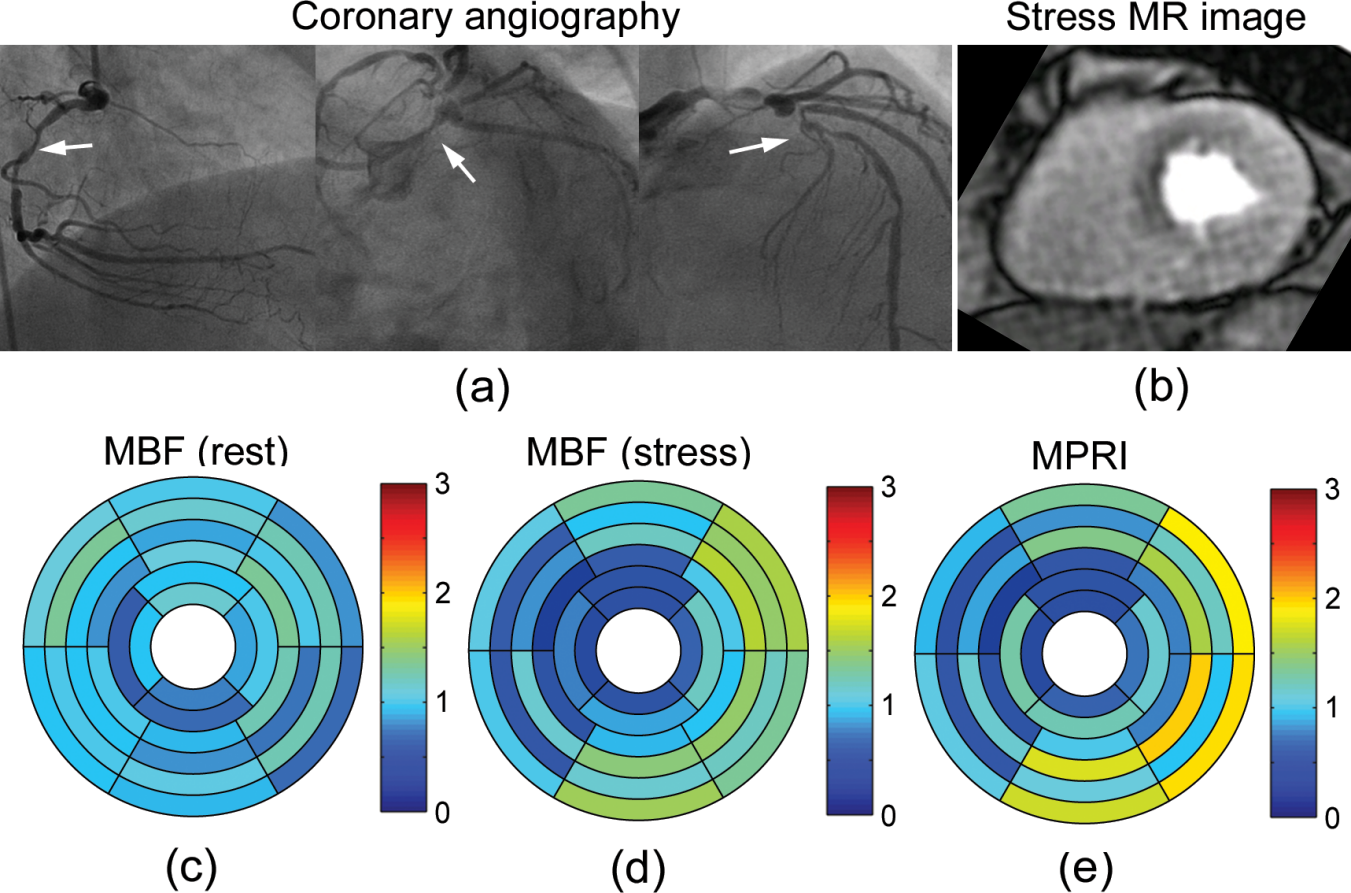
(a) Selective coronary angiography in a representative patient (65-year-old male) with severe triple-vessel disease (arrows). (b) First-pass perfusion mid-level MR image at stress showing relatively decreased flow to the anterior and anteroseptal wall. Bullseye plots of MBF (ml/g/min) (c) at rest and (d) at stress, and (e) MPRI calculated from the NTH_*f*_ method, showing more extensive perfusion abnormality.
